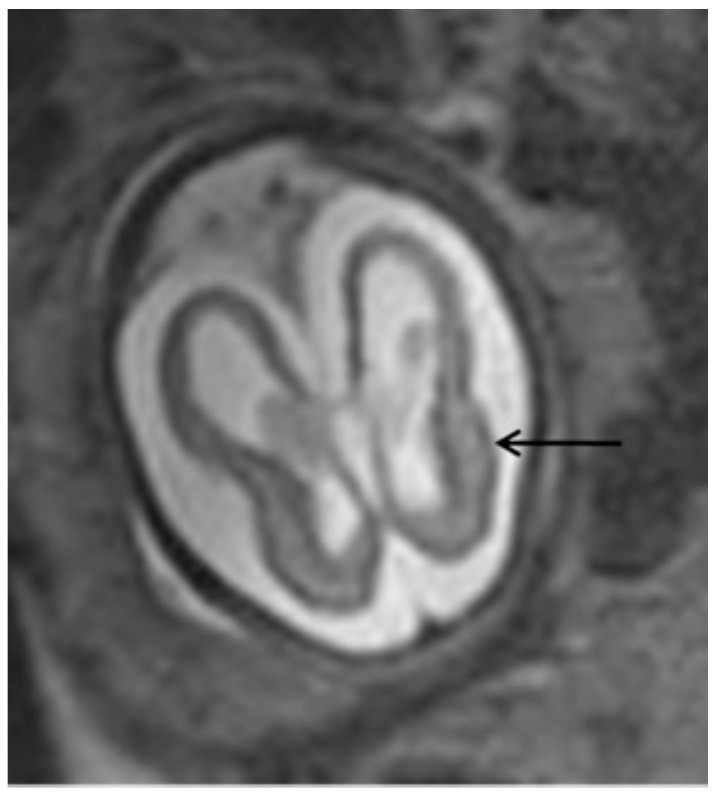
T2-weighted MRI in axial view of fetal skull showing mild ventriculomegaly and cortical atrophy (arrow).
